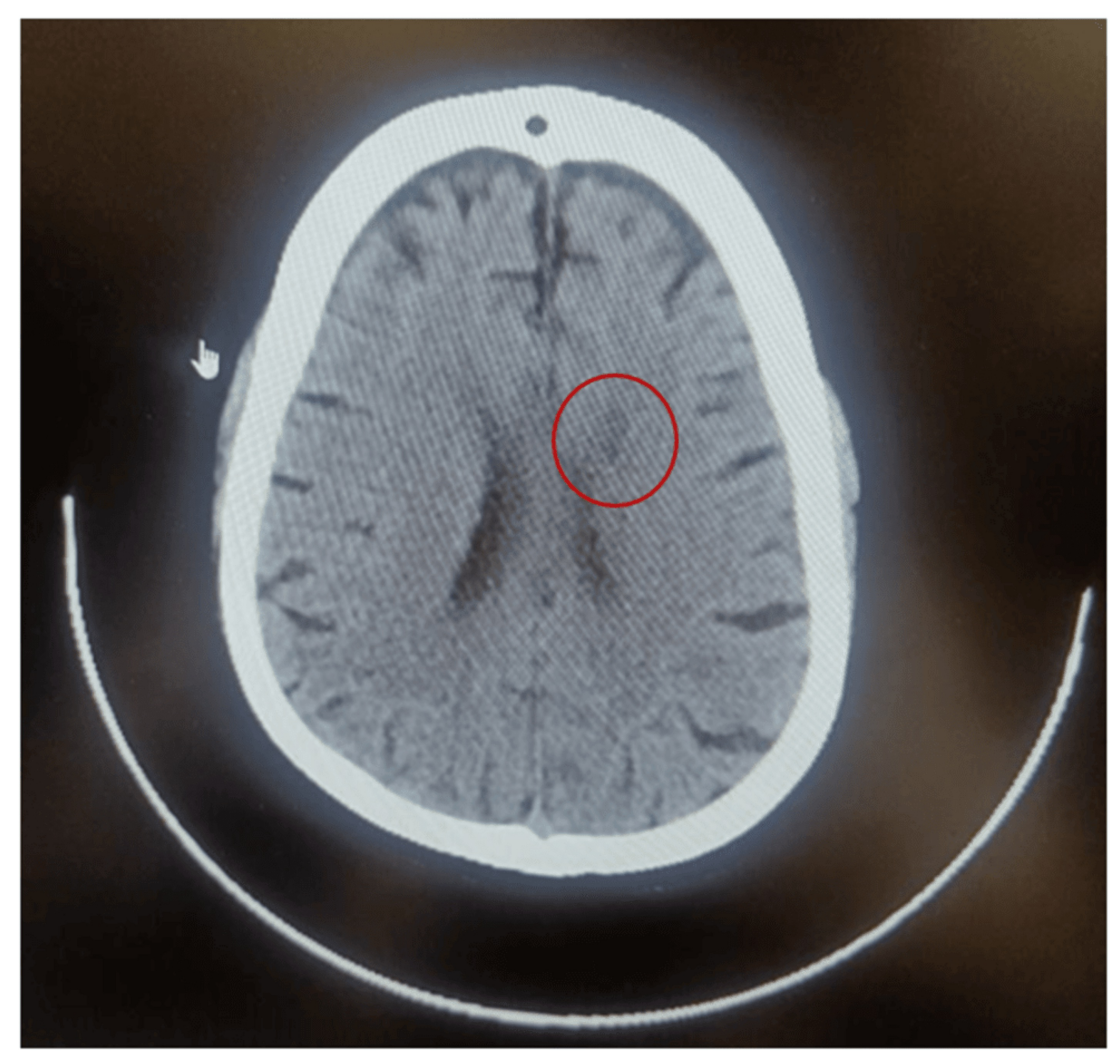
Computed tomography showing left non-hemorrhagic lacunar infarct in the frontal lobe.
